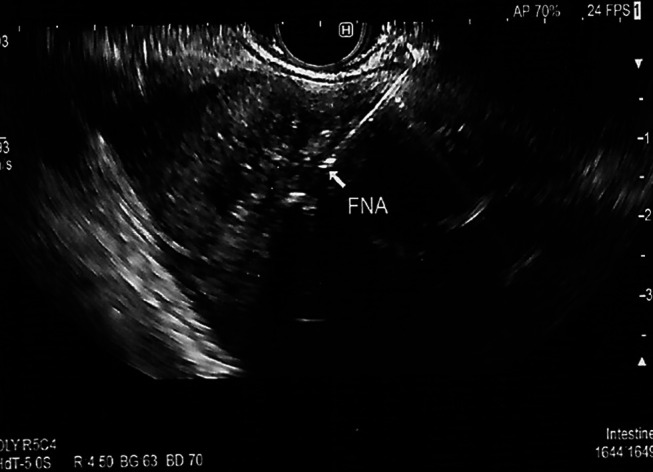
Endoscopic ultrasound-fine needle aspiration of the liver mass.
